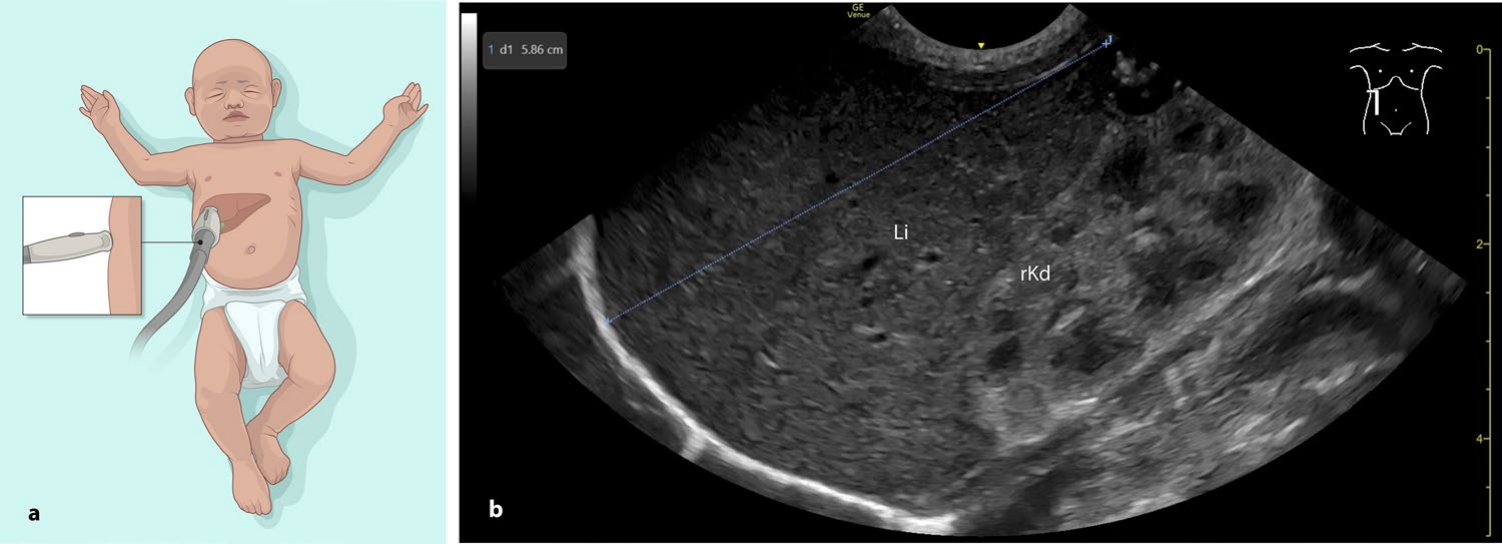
Measuring the liver length in the right AAL from the front. a infant in supine position with a convex probe positioned frontal in the right AAL (anterior–posterior plane). b sonographic craniocaudal liver length measurement. AAL: anterior axillary line, Li: liver, rKd: right kidney
